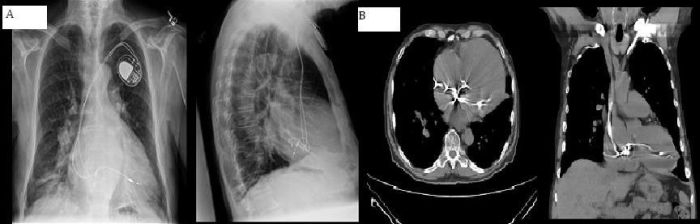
A. PA and lateral chest radiograph from a patient with inadvertent LV lead placement. The lead in the LV is clearly seen posteriorly on the lateral view. B. Chest CT from the same patient. The ventricular lead is seen passing from the RA to the LA through an ASD and from the LA to the LV through the mitral valve.
